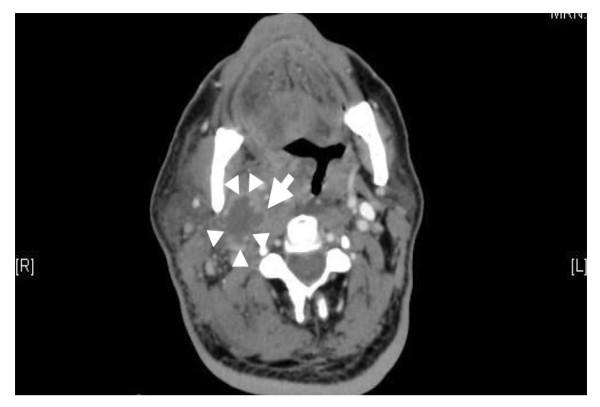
Computed tomography (CT) scan of the neck region showing the abscess content (circle of arrowheads) and thrombosis in the right internal jugular vein (single arrow).
